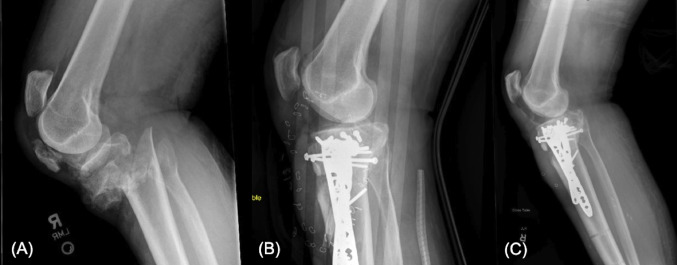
Representative case of tibial tubercle–involving tibial plateau fracture progressing to nonunion. A Preoperative lateral radiograph demonstrating displaced tubercle involvement with proximal tibial metaphyseal extension. B Immediate postoperative lateral radiograph after fixation. C Follow-up lateral radiograph showing fixation failure with persistent fragment displacement and progression to nonunion of the tubercle/metaphyseal segment. This case was later complicated by septic arthritis and hardware infection requiring irrigation and debridement with hardware removal
